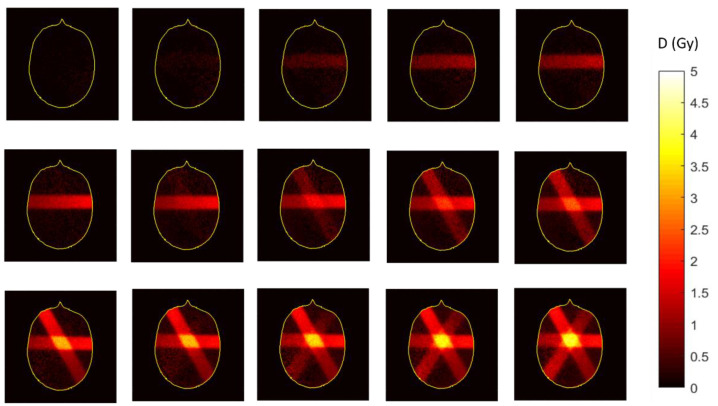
Sequential dose maps of a central slice in a MAGAT gel dosimeter recorded during treatment delivery by use of the MRI subunit on an MR-Linac [93]. The time between two adjacent images in a row is 44 s (every 4th recorded frame). Adapted from figure [93] with permission from Institute of Physics and Engineering in Medicine, Copyright 2000 IOP Publishing.
